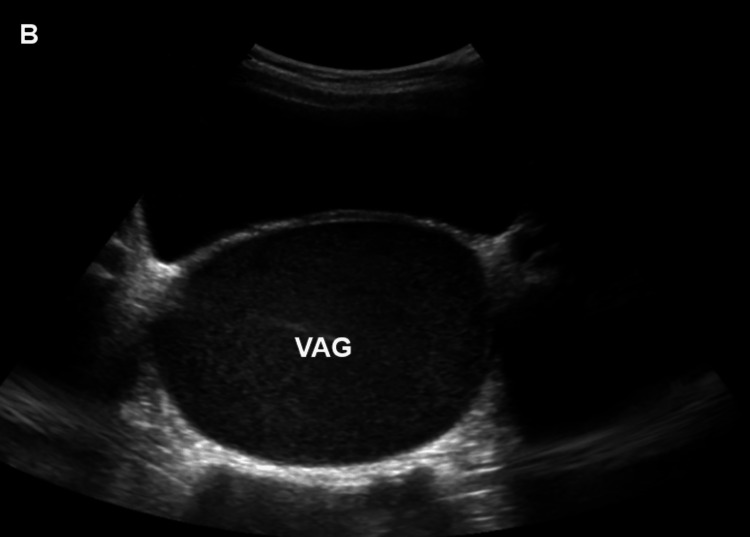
Ultrasound showing a markedly dilated vaginal canal with hypoechogenic collection
